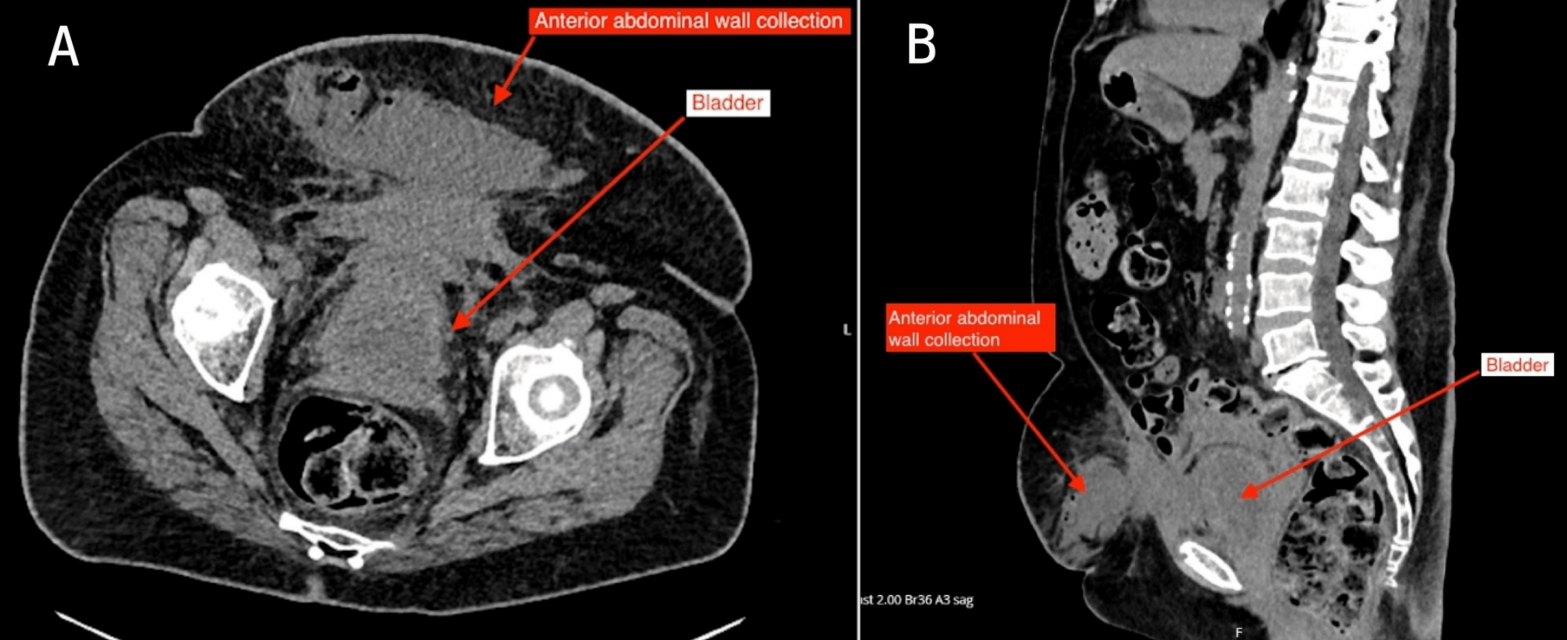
Axial (A) and sagittal (B) views of non-contrast CT abdomen and pelvis A large collection within the subcutaneous fat of the lower abdomen measuring 18 x 4.3 x 6 cm was noted. This was in continuity with the anterior abdominal wall muscles with inflammatory stranding of the intra-abdominal fat extending onto the bladder. The lack of intravenous contrast made it difficult to confirm the involvement of the anterior bladder wall CT: computed tomography
